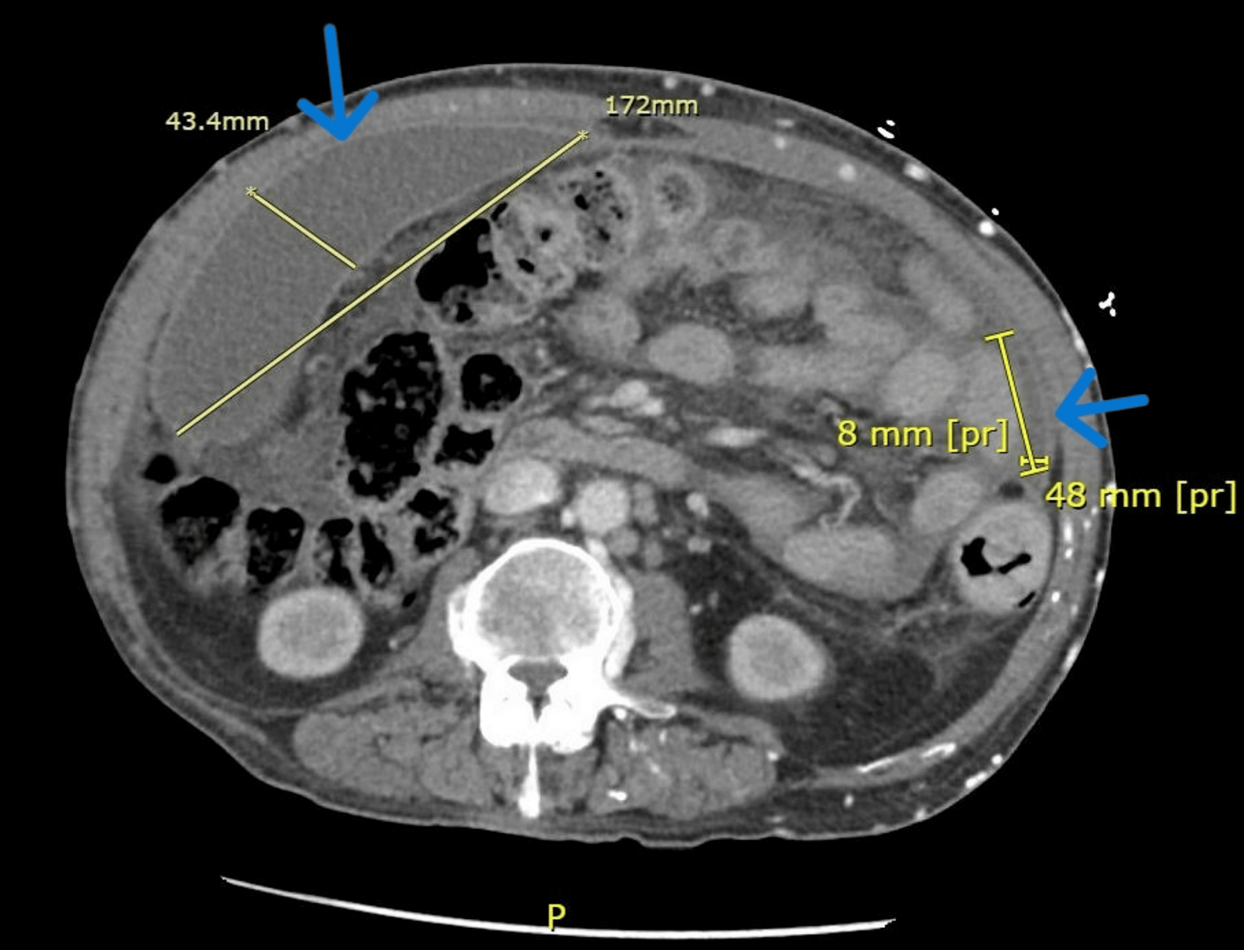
Contrasted abdominal CT scan at the patient’s readmission to the hospital. CT demonstrates a large right-sided rim-enhancing fluid collection on the left of the image. Partially imaged on the right is the most superior aspect of his smaller left-sided rim-enhancing fluid collection.
